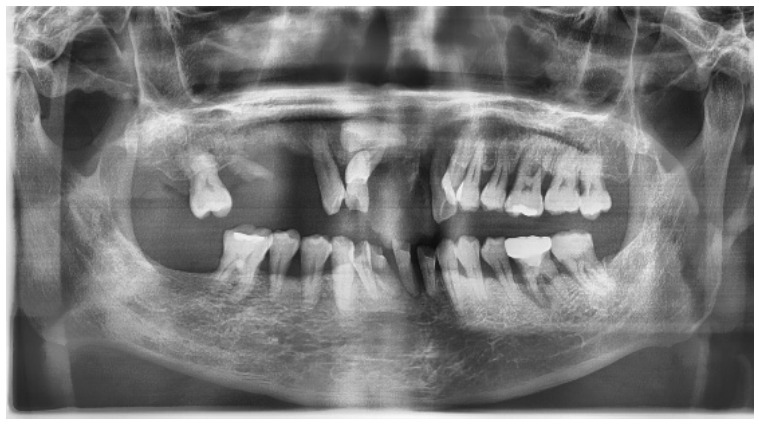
Panoramic radiography 4.5 years after the initial visit. A characteristic ground-glass appearance was not clearly identified, whereas displacement of the adjacent teeth was observed.
